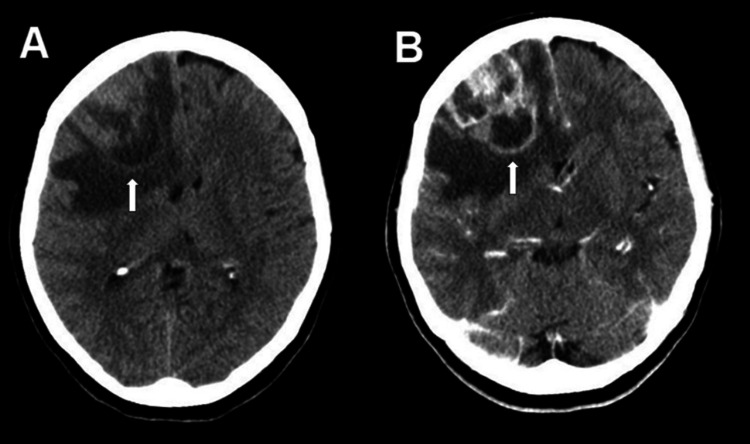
Axial CT performed because of headache revealed a large right frontal mass with perifocal edema (A) and contrast enhancement (B)
